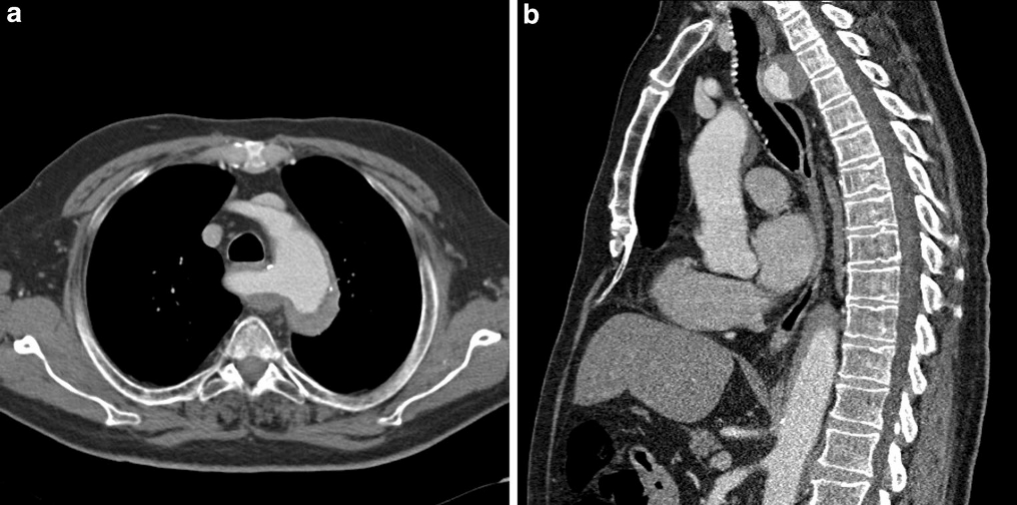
a Transversal CT section of our patient. b Sagittal CT section
